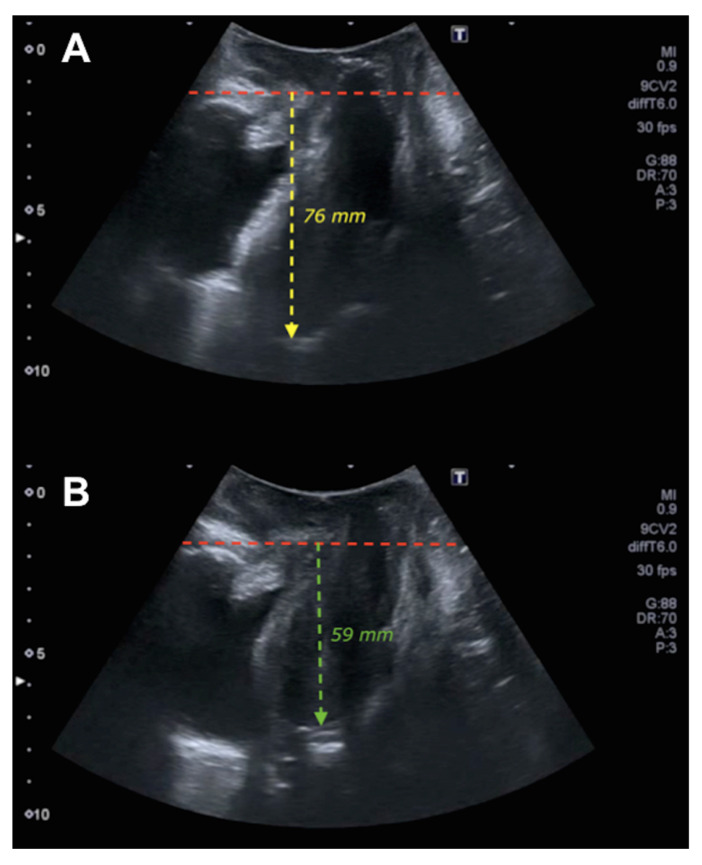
Ultrasound of uterine prolapse. Figure (A) shows the midsagittal plane of the pelvic floor at rest where the red line delimits the posteroinferior margin of the pubis and the yellow line the pubis–fundus distance at rest. Figure (B) shows the midsagittal plane of the pelvic floor in Valsalva where the red line establishes the posteroinferior margin of the pubis and the green line the pubis–fundus distance in Valsalva.
